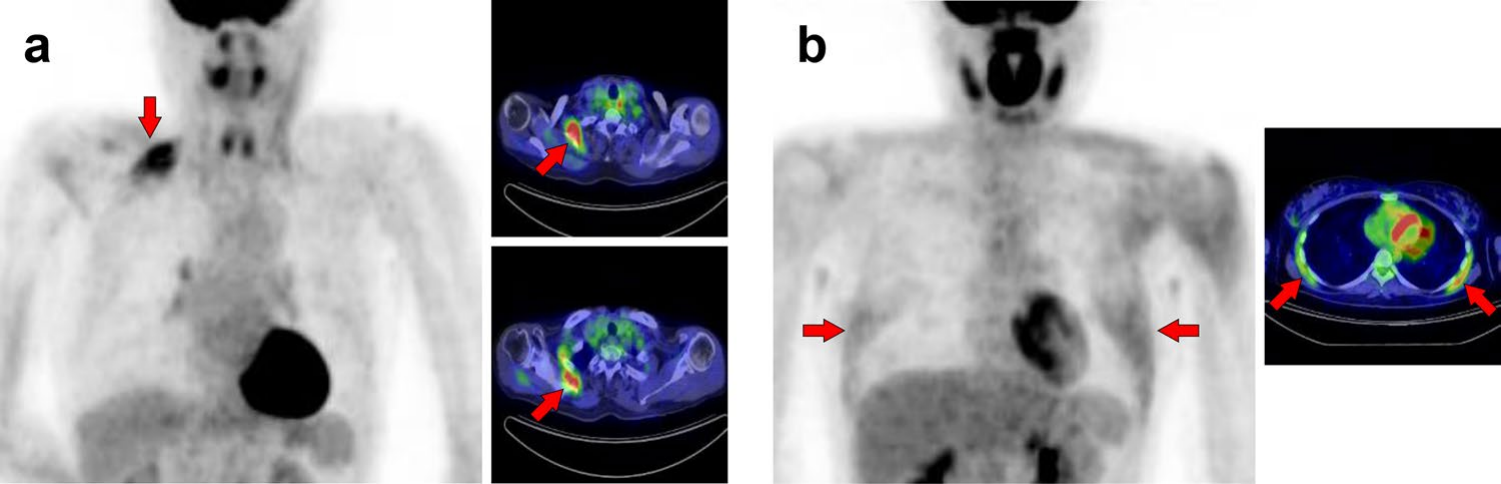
Serratus anterior muscle.* The serratus anterior muscle (a, b, arrows) can be divided into upper, middle, and lower parts. Increased uptake in the middle and lower parts observed after exercise or in hyperglycemia (b) is easy to interpret, whereas high uptake in the upper fibers alone (a) may require differentiation from malignant lesions. It is known that [^18^F]FDG accumulates in elastofibroma dorsi, which should be distinguished from muscle uptake in the serratus anterior and subscapularis muscles
